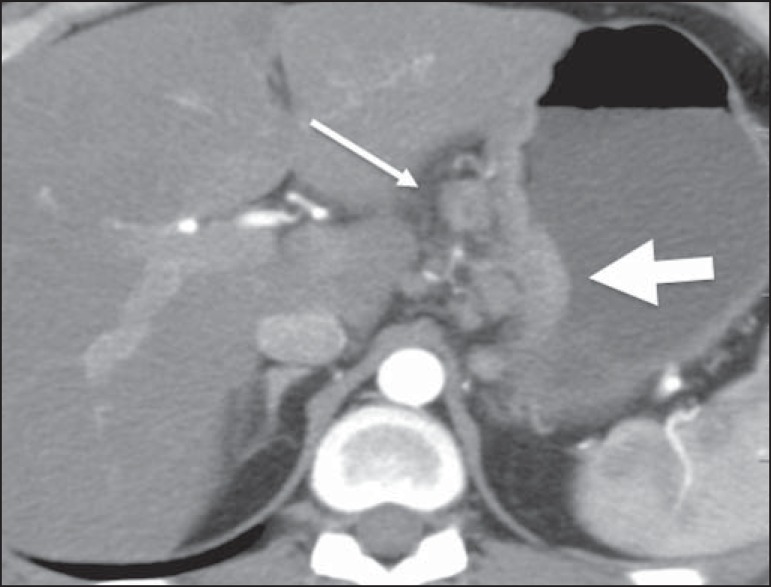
Gastric lymphoma. Axial CT of the abdomen, showing thickening of the wall
